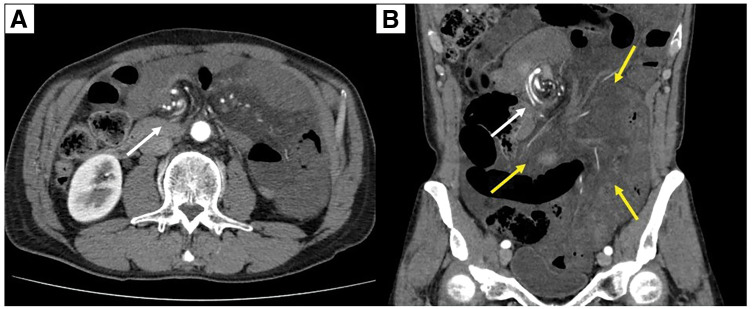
Preoperative imaging findings. (A) Axial section. (B) Coronal section. Contrast-enhanced abdominal CT demonstrates the whirl sign of a twisted mesentery (white arrows) with mild ascites. Diffuse mesenteric fat stranding is also noted (yellow arrows), with a whirl-like rotation of the mesentery and mesenteric veins around the superior mesenteric artery.
